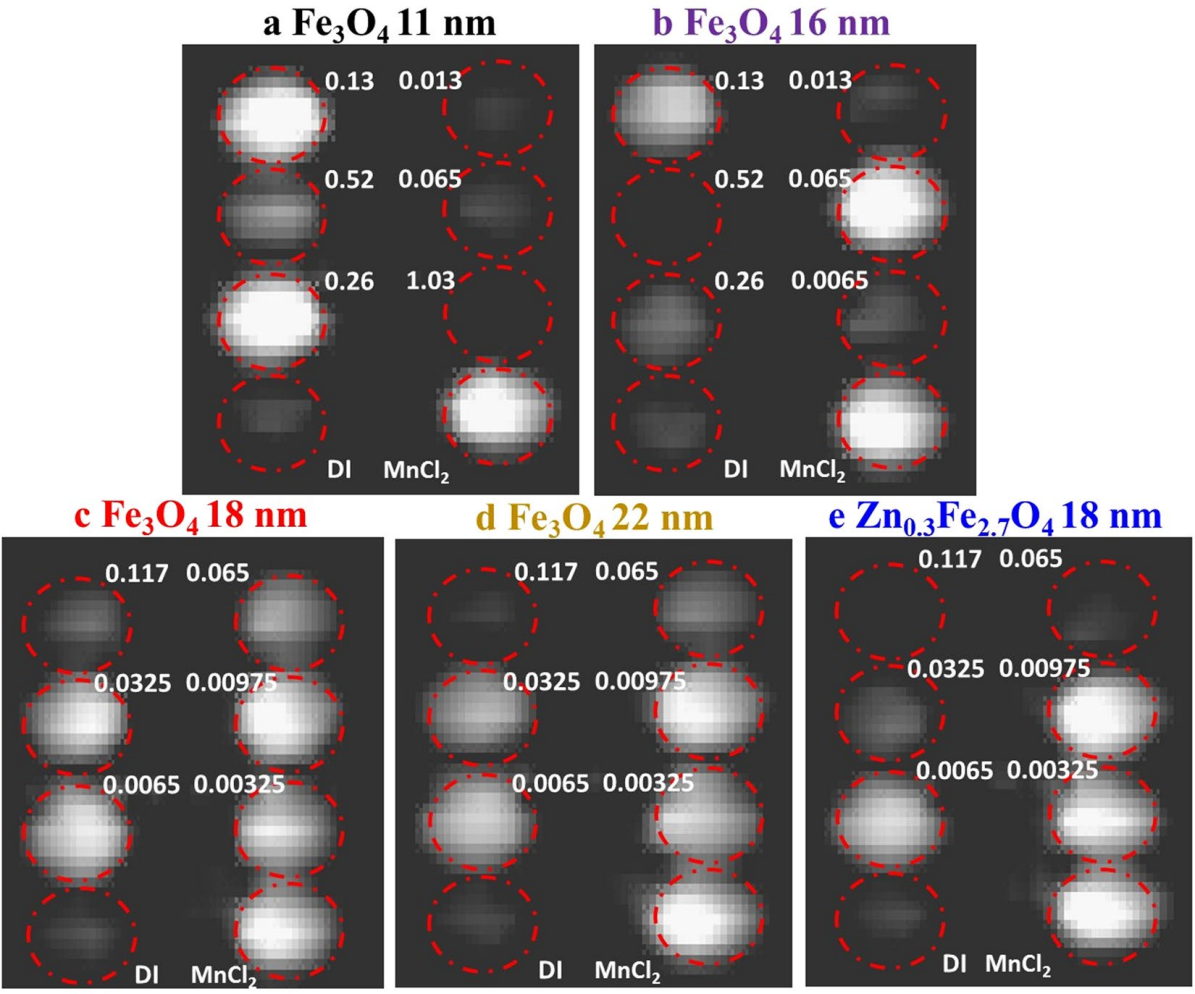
ULF MRI 2D images of different SPION concentrations in 16 mL vials with a vial diameter of 14 mm: (a) 11 nm Fe_3_O_4_ solutions, (b) 16 nm Fe_3_O_4_ solutions, (c) 18 nm Fe_3_O_4_@SiO_2_ solutions, (d) 22 nm Fe_3_O_4_@SiO_2_ solutions, and (e) 18 nm Zn_0.3_Fe_2.7_O_4_@SiO_2_ solutions. The Fe concentrations of the solutions (in mM) are listed in the figures. DI water and MnCl_2_ (0.167 mM Mn in DI water) control samples are placed at the bottom of each image for normalization and comparison.
